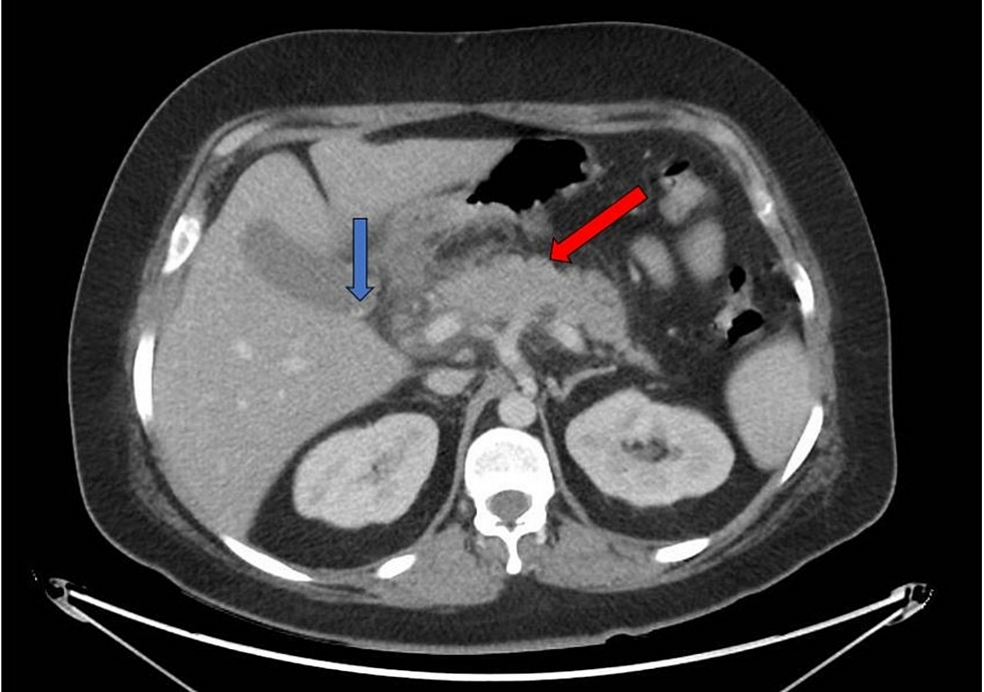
CT scan of the abdomen. The red arrow indicates the edematous pancreatic body and head with moderate surrounding peripancreatic inflammatory stranding and edema suggestive of acute pancreatitis. The blue arrow indicates a small gallstone visualized in the gallbladder. CT: computed tomography
